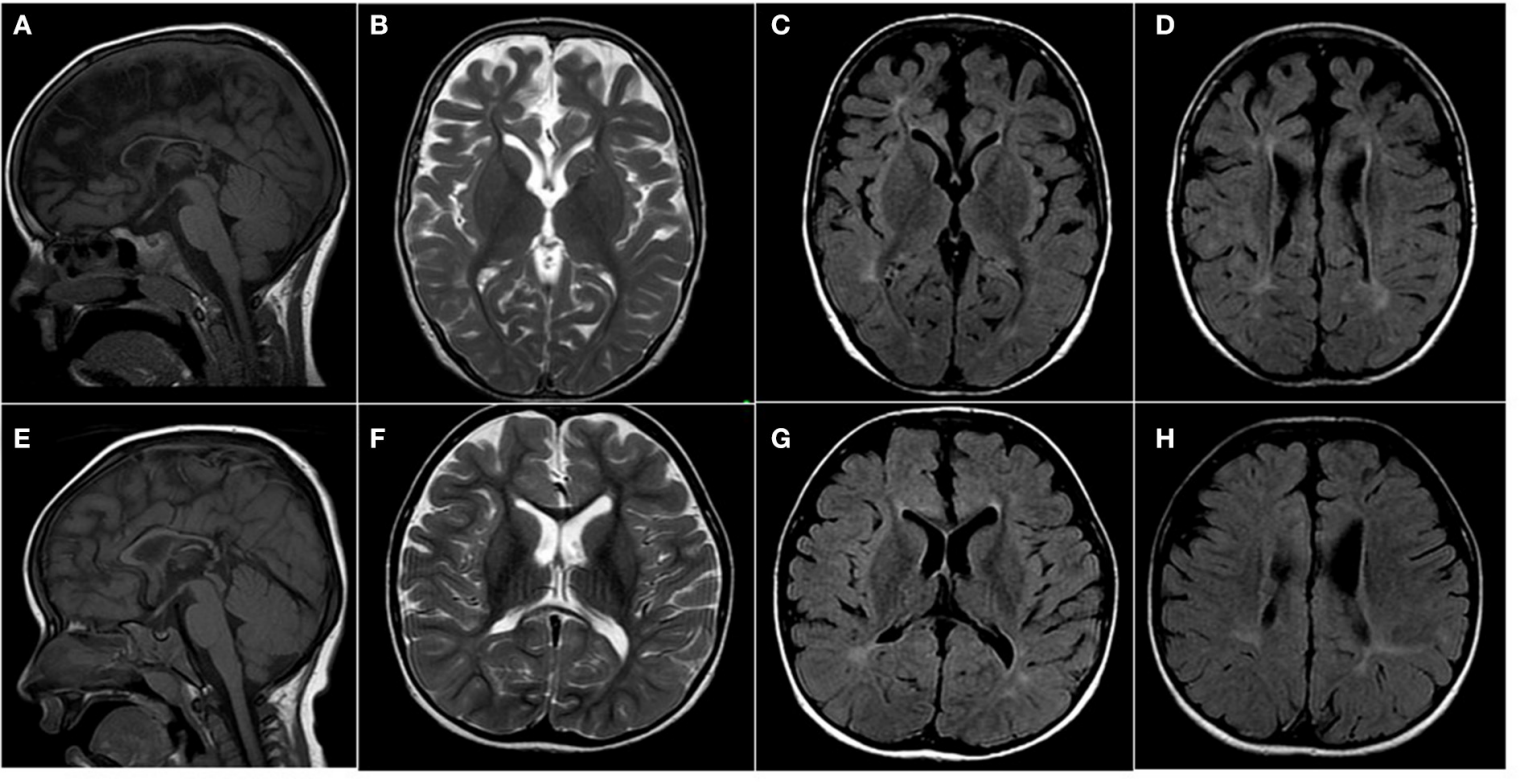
Brain imaging of subjects with PLPHP deficiency. MRI of subjects 2 (A–D) and 3 (E,F) at 4.5 and 2.5 years. (A,E) Sagittal T1 demonstrates thin corpus callosum. (B,F) Axial views show a patchy bilateral increase in T2 and (C,D,G,H) FLAIR signal in the deep white matter around the ventricles at bilateral frontal and bilateral peritrigonal regions. Both subjects have cerebral atrophy, most noted in subject 2, with a prominence of CSF spaces, particularly over frontal and sylvian regions bilaterally.
